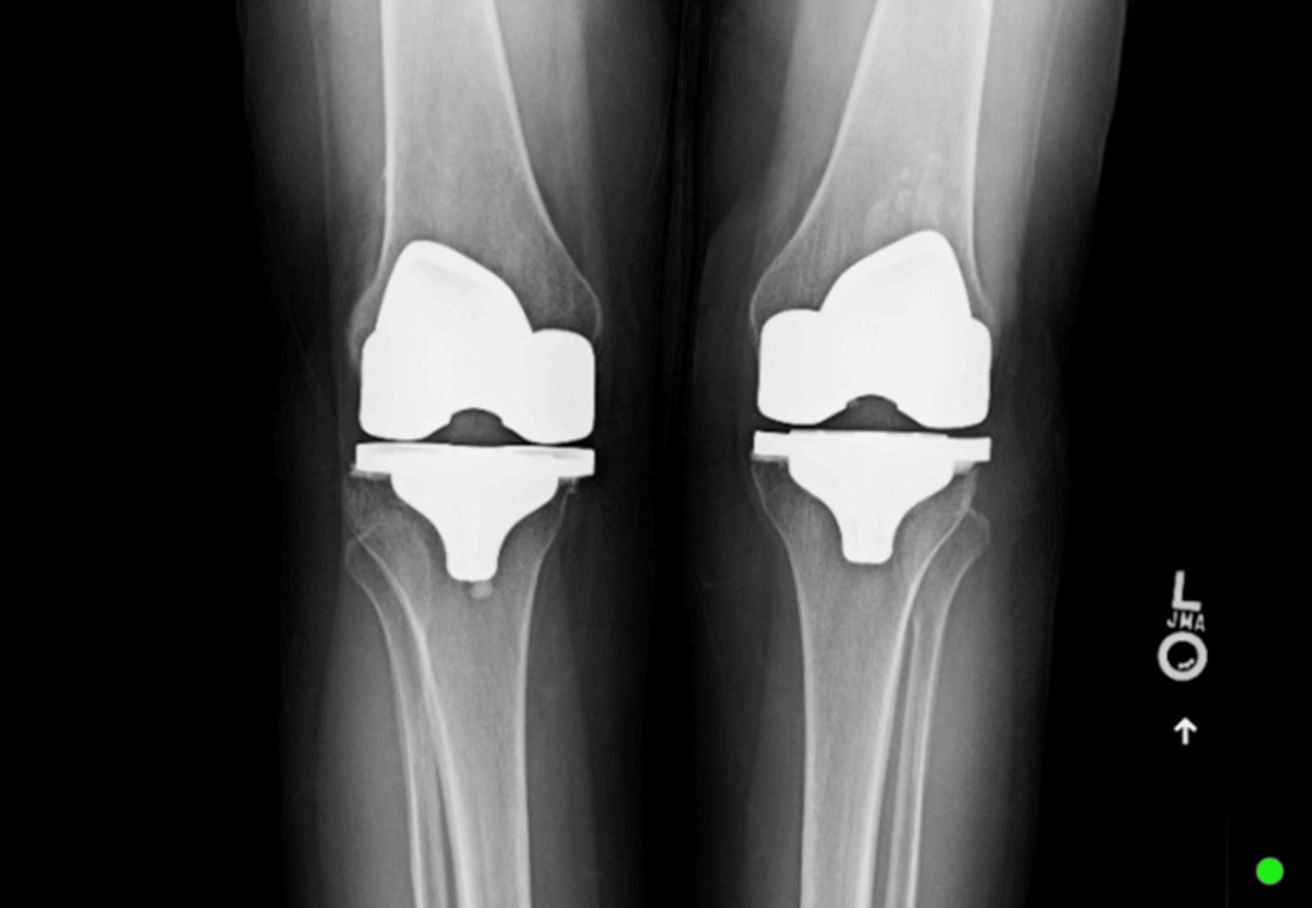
Preoperative anteroposterior radiograph of the bilateral knees
